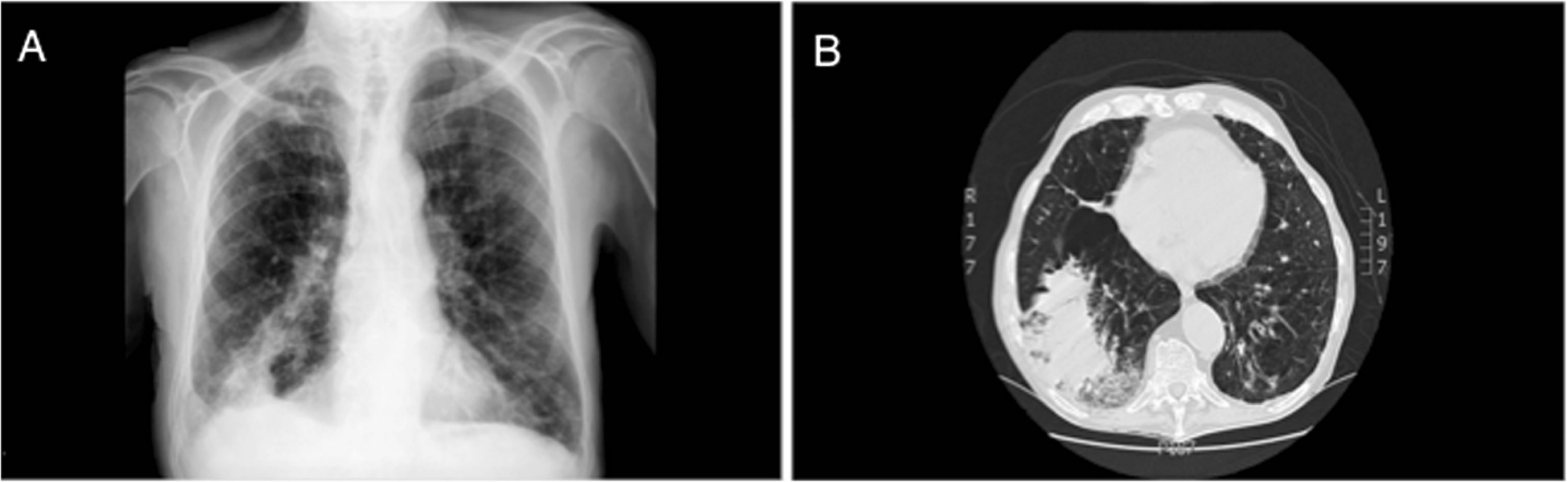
a Posteroanterior chest x-ray of the right lower lobe with alveolar infiltrate and a reticular interstitial pattern at the lung bases. b Chest computed tomographic scan showing right lower lobe alveolar consolidation with minimal pleural effusion, severe bilateral diffuse emphysema, and signs of chronic bronchitis.
